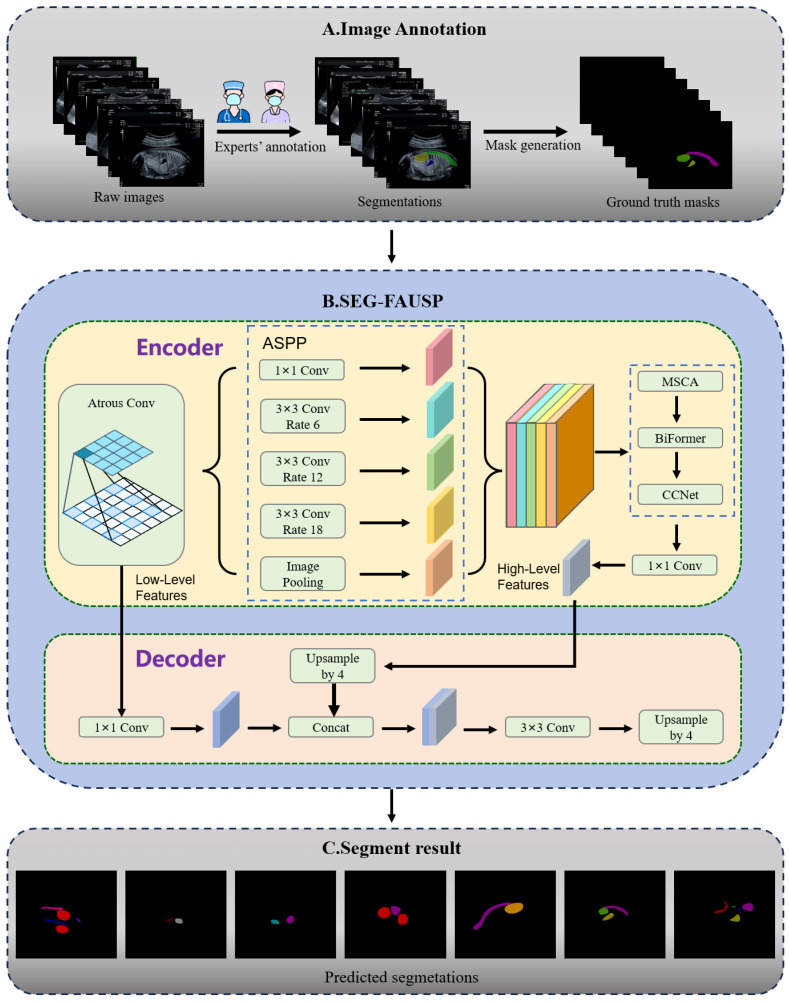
Overall workflow of the proposed SEG-FAUSP framework. First, fetal abdominal ultrasound images are collected and annotated by clinical experts to generate pixel-level masks of key anatomical structures. The images are then used to train the proposed SEG-FAUSP network. The network performs semantic segmentation to identify multiple anatomical structures simultaneously.
